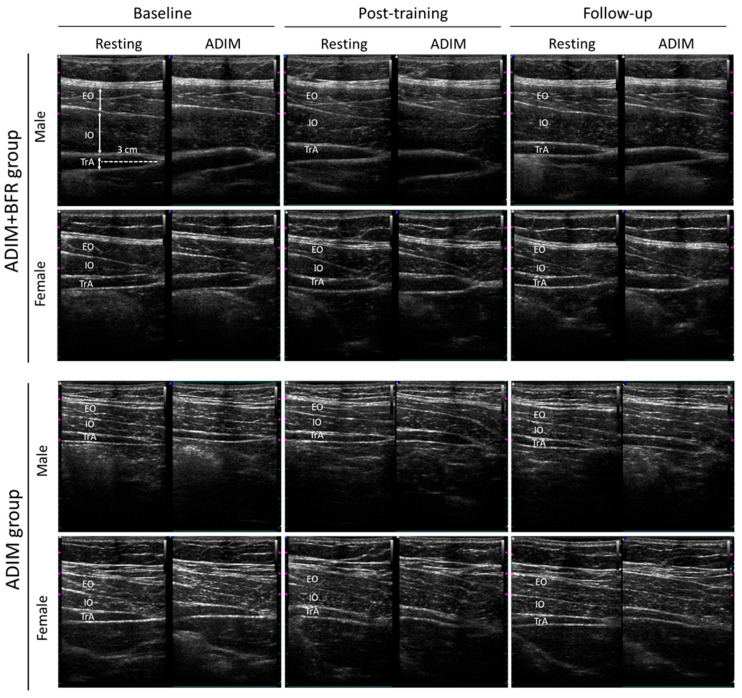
Ultrasound images of muscle thickness change across different time points and interventions. Changes in external oblique (EO), internal oblique (IO), and transversus abdominis (TrA) muscle thickness are shown through ultrasound imaging at baseline, post-training, and at follow-up during both resting and ADIM states. Specifically, the ADIM + BFR intervention significantly increased TrA thickness in both male and female participants.
